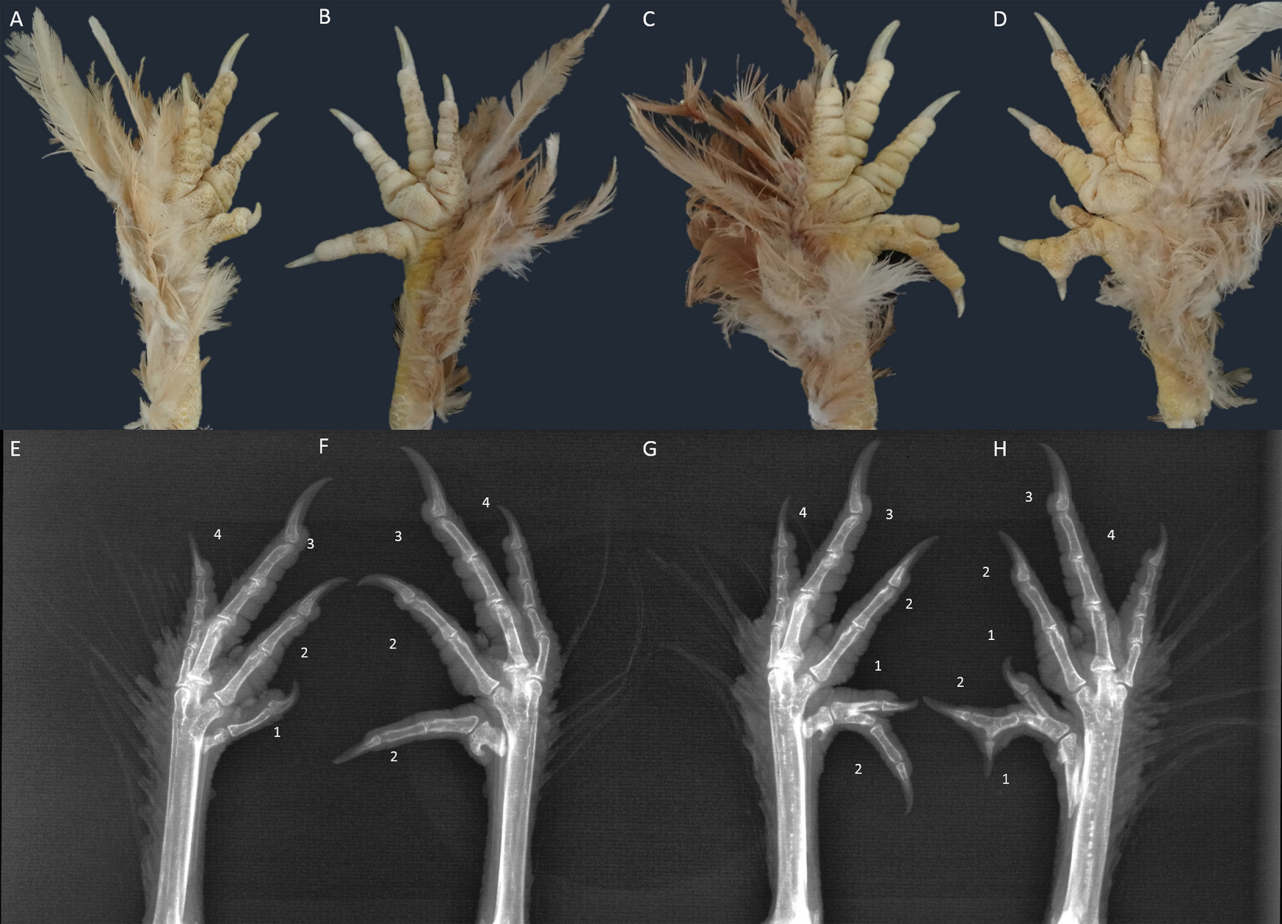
Variation in X-ray digital radiography with respect to Beijing-You foot polydactyly. A-D show the different foot phenotypes of Beijing-You chicken, E-H show the X-ray digital radiography coordinately with A–D. A & E: non-polydactylous foot with four digits, identified from anterior to posterior (1, 2, 3, 4). B & F: Beijing-You foot with four digits, with a polyphalange in digit “1” making it digit “2” (2, 2, 3, 4). C & G: polydactylous Beijing-You foot with five digits (2, 1, 2, 3, 4). D & H: polydactylous Beijing-You foot with six digits (1, 2, 1, 2, 3, 4).
